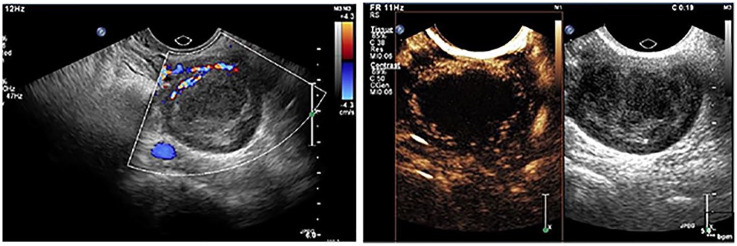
Subserosal fibroid (image made at our own institution). On the left CEUS image obtained 19 seconds after contrast injection, shows peripheral enhancement without enhancement in the central part of the fibroid. On the right conventional gray-scale ultrasound image of the same fibroid.
